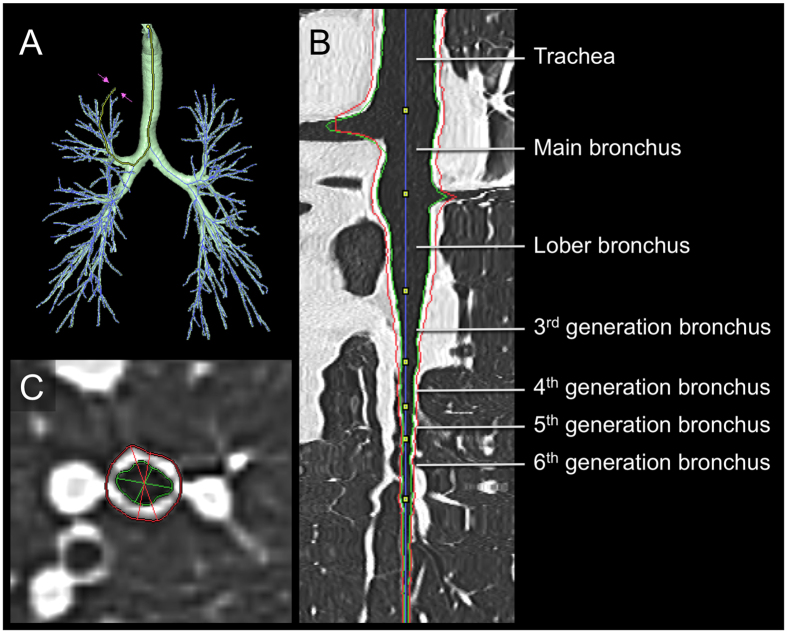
Three-dimensional CT analysis of the airway. (A) The image of the airway tree reconstructed by three-dimensional computed tomography. (B) The longitudinal section of the reformatted airway in the multiplanar reconstruction image. (C) Measurement of the airway in the short axis image. Red and green lines indicate the outer and inner edge of the airway, respectively.
